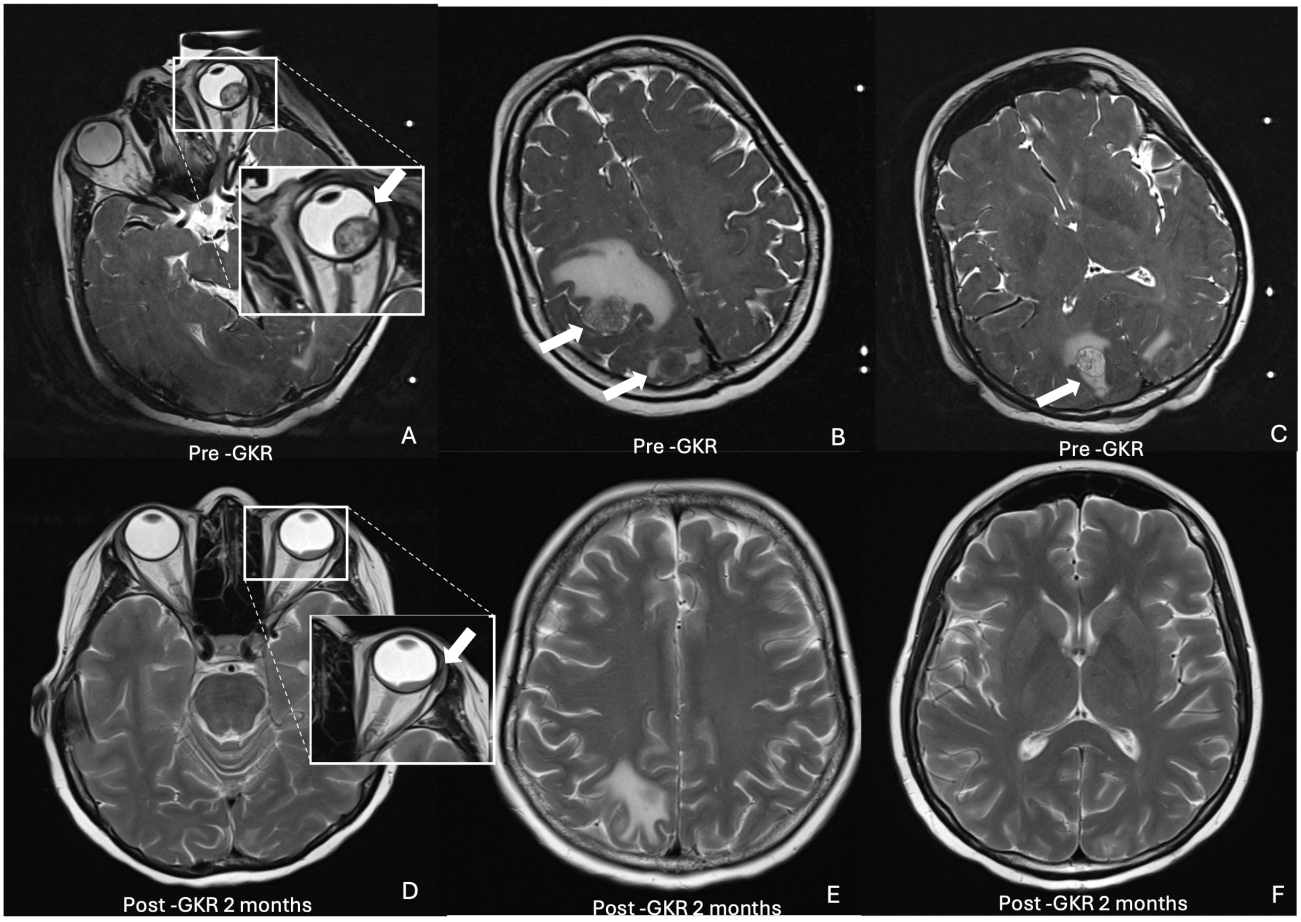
Axial T2-weighted MRI brain and orbit showing choroidal and multiple intracranial metastatic lesions pre (top row) and post GKR 2 months (bottom row). (A) inset. Intraocular mass (arrow) at superolateral left globe. (B) Metastatic nodules with prelesional oedema (arrows) at right parietal and occipital lobes (C) Metastatic nodule (arrow) at right occipital lobe. (D) inset. Reduced size of intraocular mass (arrow). (E) Reduced and resolved intracranial nodules at right parietal and occipital lobes. (F) Resolved nodules at occipital lobe.
